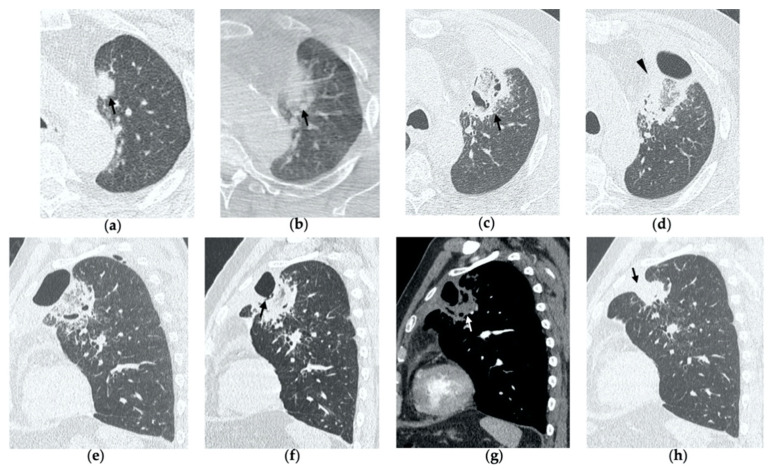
68-year-old man with a biopsy-proved squamocellular carcinoma in the left upper lobe. (a) Axial CT before treatment. (b) Cone-beam CT image at the end of MWA shows a wide GGO area (black arrow) among the ablation site. (c–e) Since persistent hydro-pneumothorax on chest X-rays is detected, CT without contrast administration is performed before the usual 1-month follow-up; axial and sagittal CT images show a large consolidation with central cavitation (black arrow); on a different level, a concomitant hydro-pneumothorax is evident (arrowhead); the diagnosis of broncho-pleural fistula is made. (f,g) Sagittal 2-month follow-up CT images show a decrease in size of the cavitated consolidation and hydropneumothorax; a communication between a bronchus and the pleural cavity is evident (black arrow); (f) the consolidation shows no contrast enhancement (white arrow). (h) Axial 4-month follow-up CT image shows a decrease in size of the consolidation with complete resolution of both cavitation and pneumothorax (black arrow).
